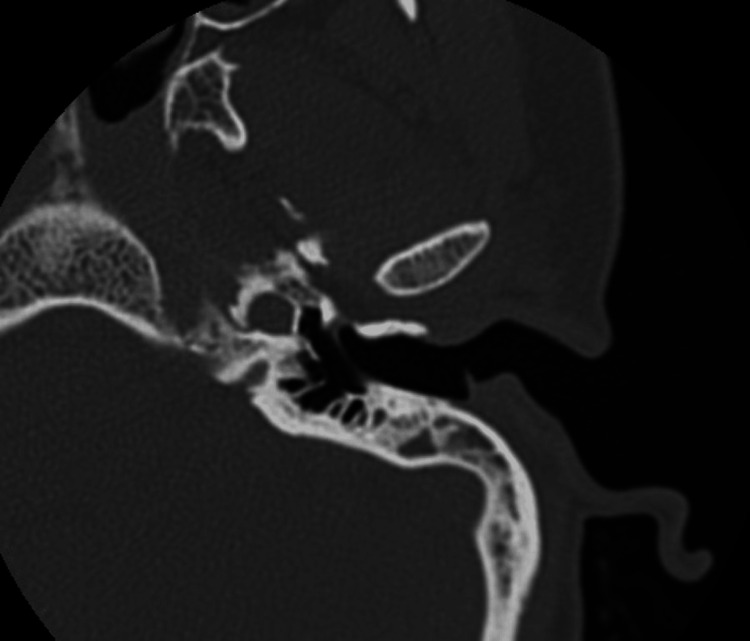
Sample error image of a misclassified SCOM CT scan image using the kNN model SCOM: suppurative chronic otitis media; CT: computed tomography; KNN: k-Nearest Neighbors.
